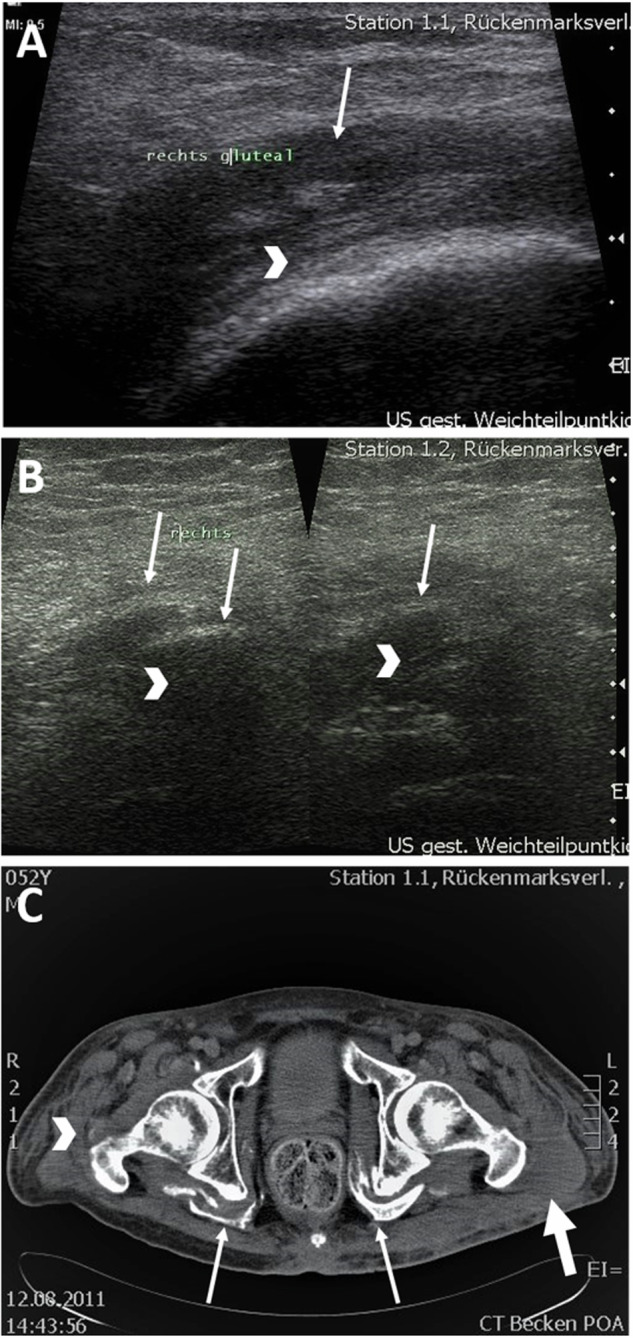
Sonographic images and computed tomography. A Sonographic image. Participant 4: edematous altered gluteal muscles (arrow) in the area of the greater trochanter (arrowhead). B Sonographic image. Participant 4: Calcifications (arrow) with dorsal sound extinction (arrowhead). C Computed tomography of the pelvis. Marked NHO in the area of the deep hip muscles (arrow) and incipient NHO in the area of the trochantor major as well as M. obturatorius on the right (arrowhead). Muscular edema (thick arrow).
